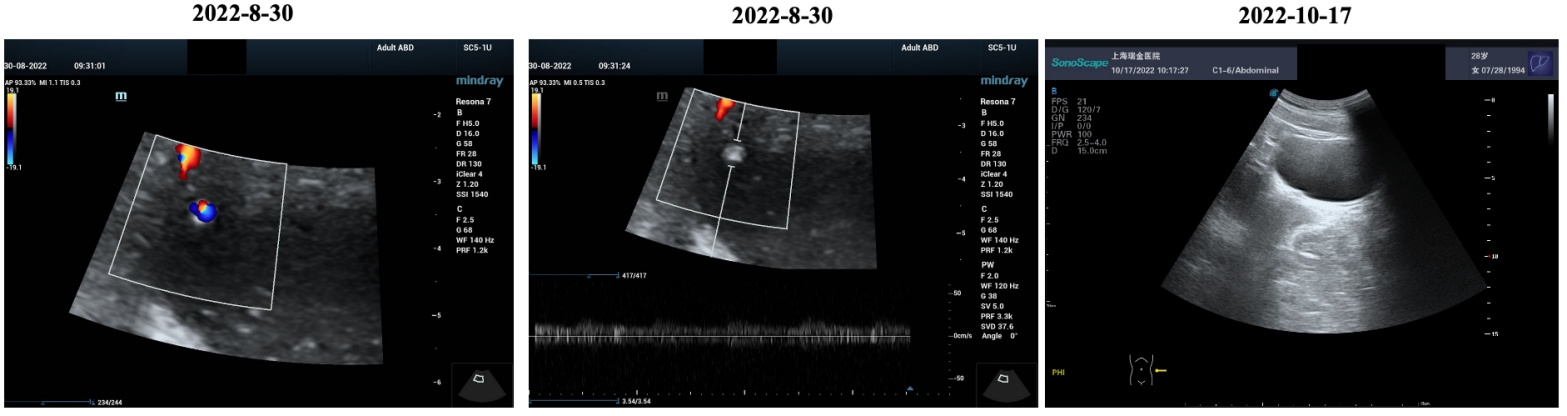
B-mode ultrasonography indicated a high echo in the right lateral wall of the bladder.
